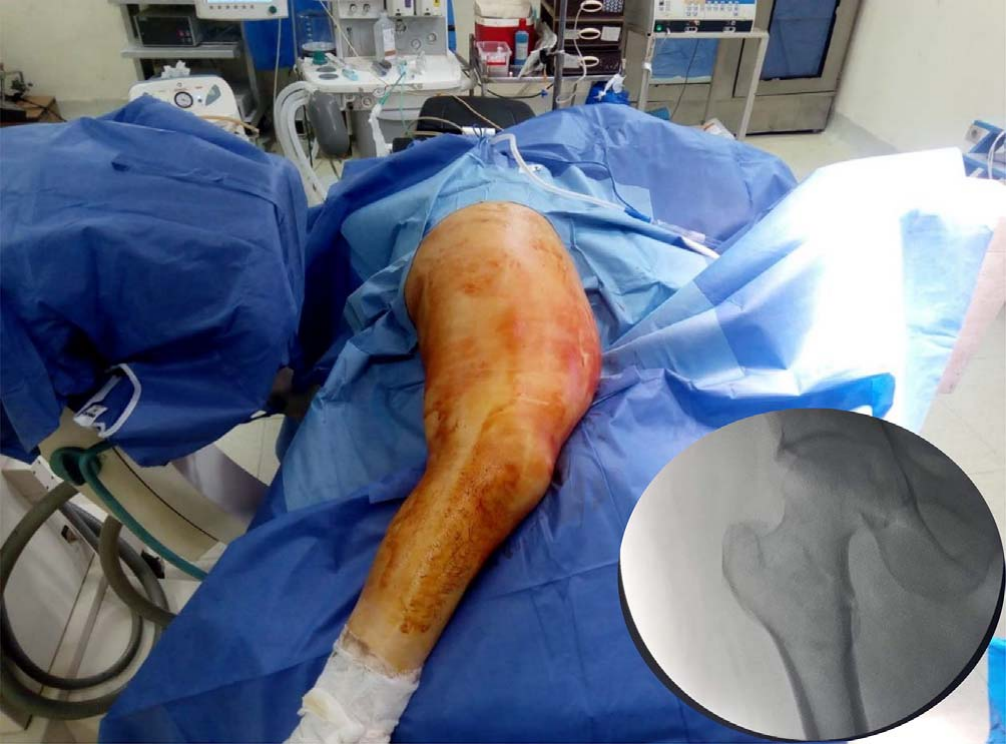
The position of the patient in the lateral decubitus position after draping the patient and the C-arm. The bottom left photo shows the obtained X-ray without traction.
